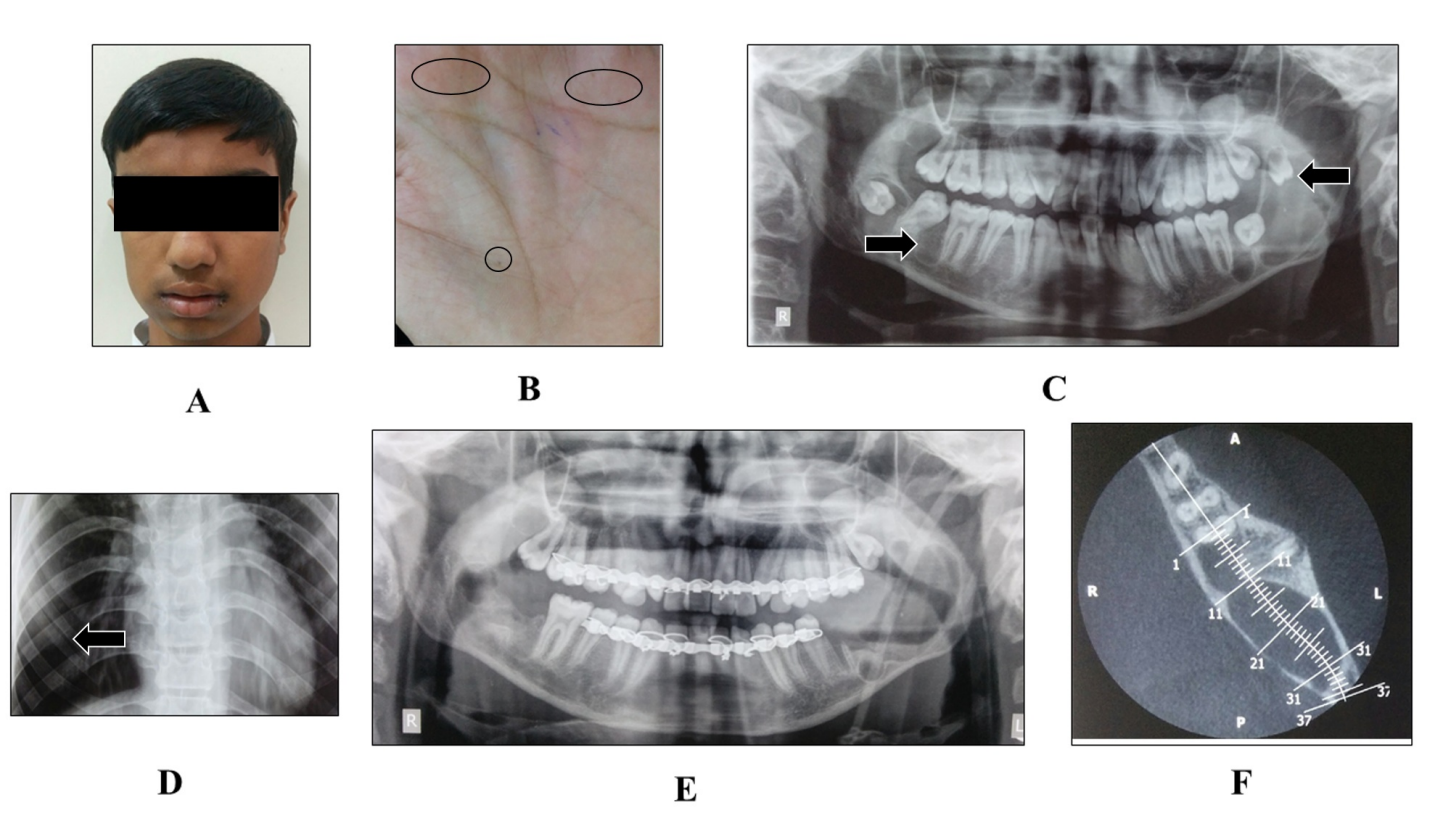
(A) Extraoral profile; (B) General examination showing palmar pits; (C) Panoramic radiograph; (D) Chest radiograph; (F) Cone beam CT scan (axial view); (E) Postoperative panoramic radiograph
